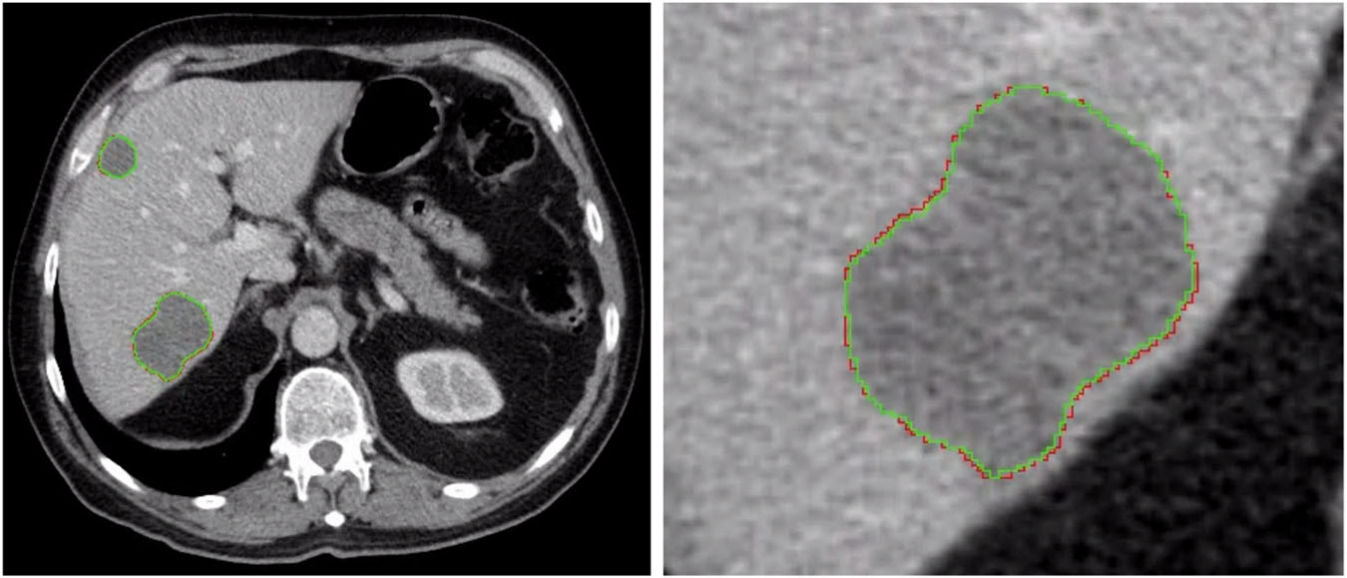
Comparison between the COALA model’s segmentation and the merged segmentation within a portal venous phase axial computed tomography scan. Red = automatic segmentation performed by our model, green = merged manual segmentation performed by three radiologists and merged using the STAPLE algorithm
